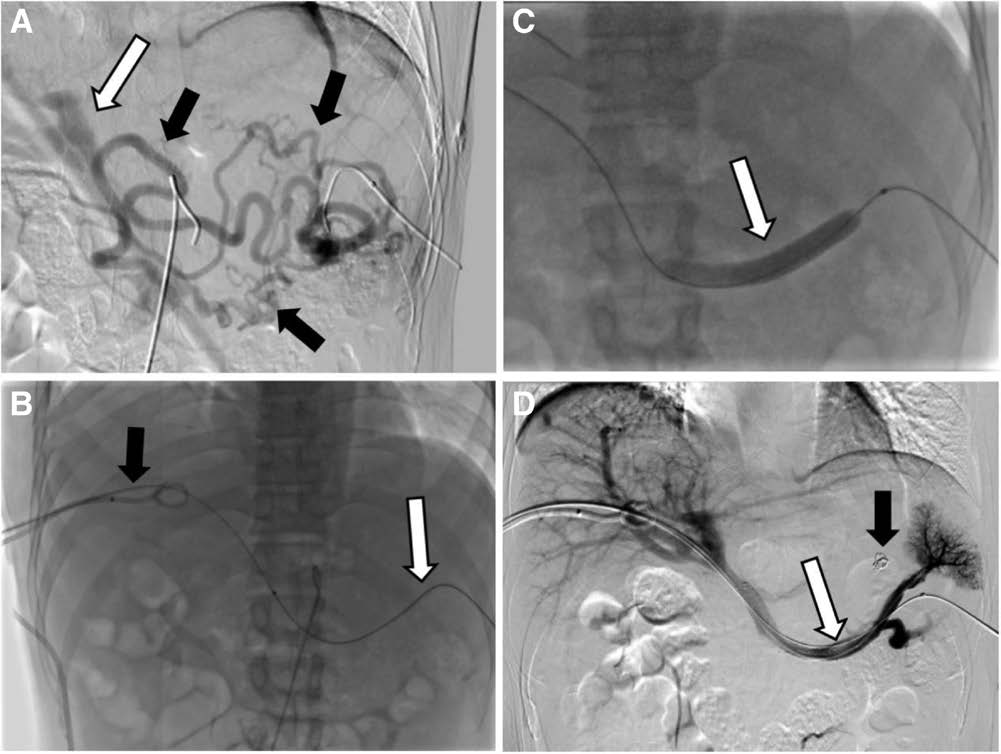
A 49-year-old male underwent percutaneous dual approach splenic venoplasty due to LSPH. (A) Under the guidance of DSA and ultrasound, percutaneous puncture of the inferior splenic vein was performed and a guide wire catheter was inserted. The splenic venography showed no visualization of the main splenic vein, while the gastric omental vein and surrounding collateral vessels (black arrow) showed no visualization and flowed back to the portal vein (white arrow); (B) the guide wire catheter was used to explore the splenic vein through the splenic puncture pathway and successfully passed through the narrow segment of the splenic vein (white arrow). An 8F long sheath was introduced into the portal vein puncture pathway to grasp the tip of the guide wire and pull it out (black arrow); (C) introduce 4, 6, and 8 mm balloon catheters from the portal vein puncture pathway through a penetrating guide wire to dilate the splenic vein occlusion segment (white arrow); (D) implant 10 mm × 8 cm, 12 mm × 8 cm vascular stent and 10mm balloon catheter were introduced for stent dilation. The splenic vein system was reimaged to show that the splenic vein had been opened (white arrow), and the steel ring used in the embolization of gastric coronary vein and gastric varicose vein (black arrow) was visible. DSA = digital subtraction angiography, LSPH = left-sided portal hypertension.
